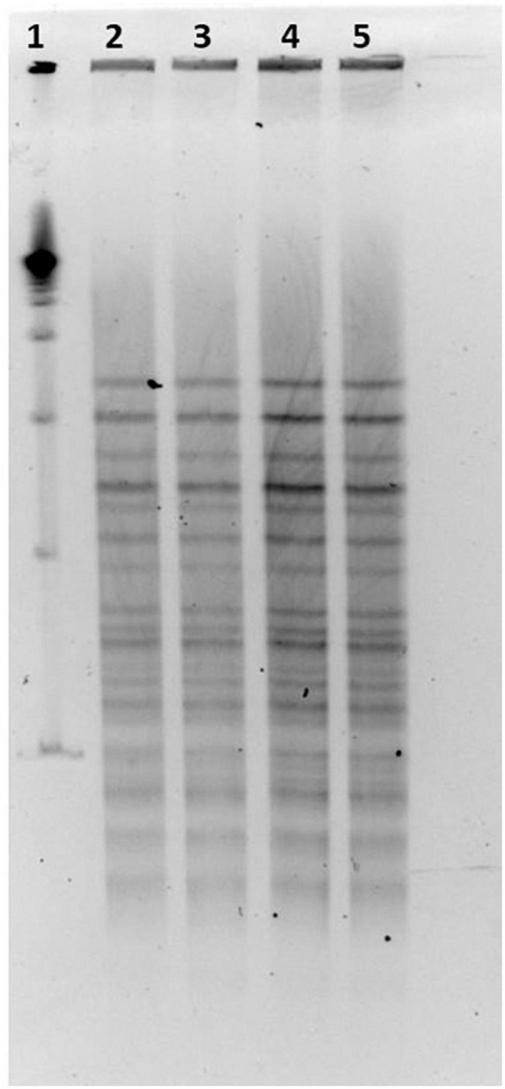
(1) LP-CT strain PFGE profiles with *Not*I; 19 marker; (2) LP-CT 1° step at 30°C; (3) LP-CT freeze-dried at 30°C; (4) LP-CT 1° step at 37°C; and (5) LP-CT freeze-dried at 37°C.
